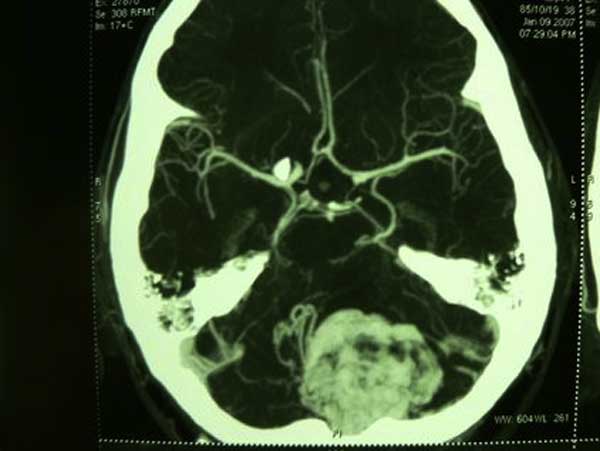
Computed tomographic angiogram of brain showing a highly vascular mass supplied by multiple tortuous feeders originating from cerebellar cortex as well as postero-inferior cerebellar artery.
